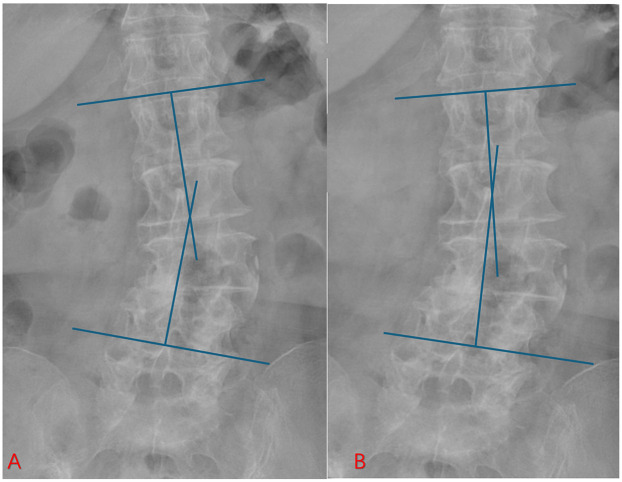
Preoperative and postoperative radiographs of an elderly patient with degenerative scoliosis. (A) Preoperative lumbar x-ray showing coronal deformity with degenerative changes. (B) One-year postoperative follow-up radiograph after PEID. The coronal Cobb angle remained stable without progression of scoliosis, indicating that the PEID effectively relieved symptoms without accelerating deformity progression.
